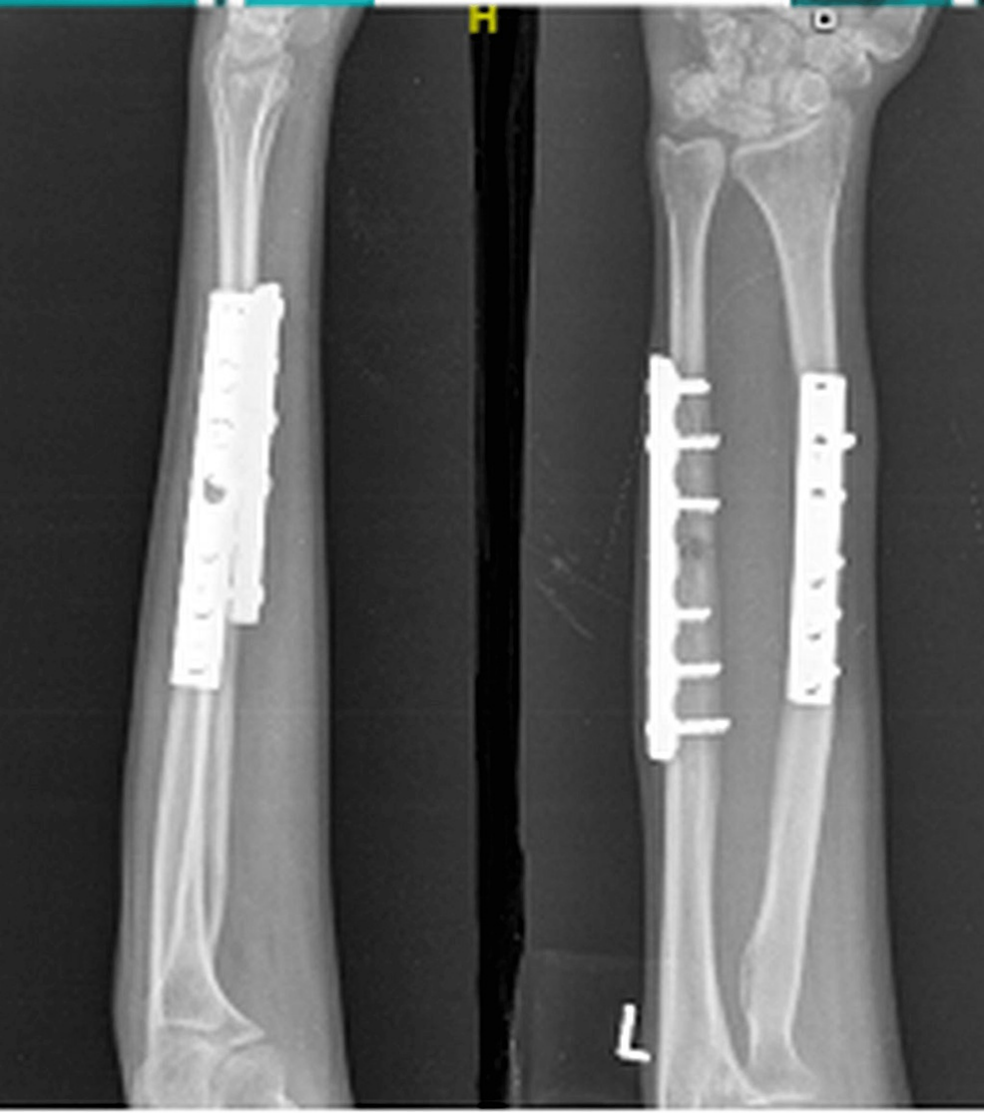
Postoperative follow-up X-ray of the patient.
